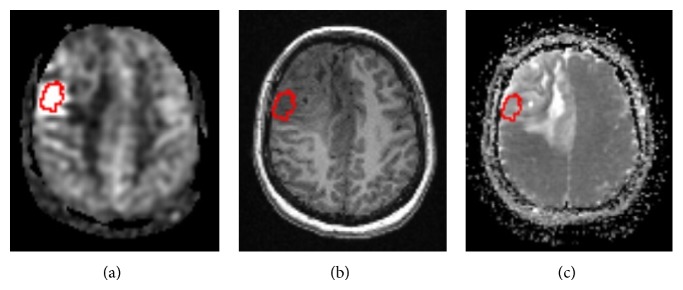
Coregistered images with ROIs. CBF map (a) and corresponding slice of 3D-T1WI (b) as well as DWI map (c).
